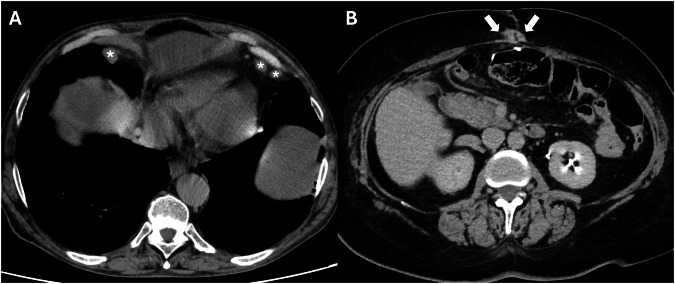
CT porta-venous phase, axial plane, showed the two most relevant red flag findings in subphrenic nodes (A) (stars) and subcutaneous implants in the periumbilical area are known as “Sister Mary Joseph’s nodules” (B) (arrows)
